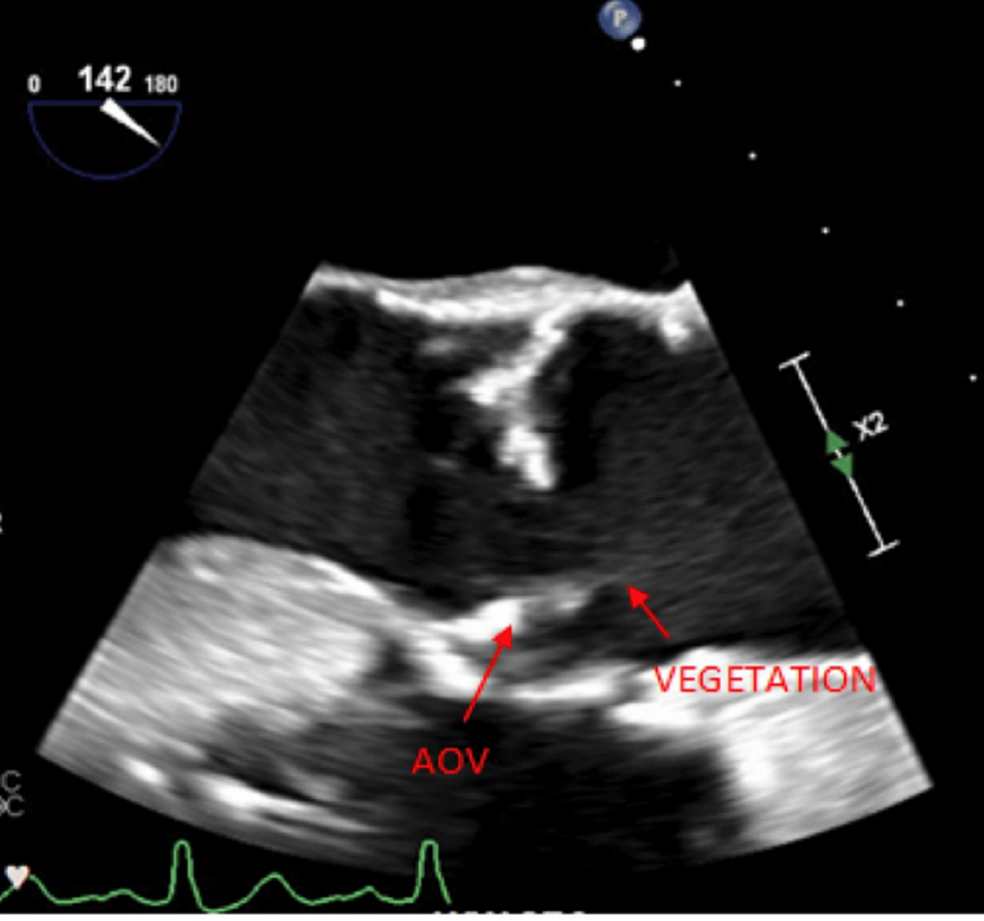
Remnant of aortic valve vegetation after antibiotics Transesophageal echocardiogram post-IV antibiotics showing minimal strands of vegetation. AOV: aortic valve; LVOT: left ventricular outflow tract; SINUS: sinus of Valsalva; STJ: sino tubular junction; ASC AO: ascending aorta
